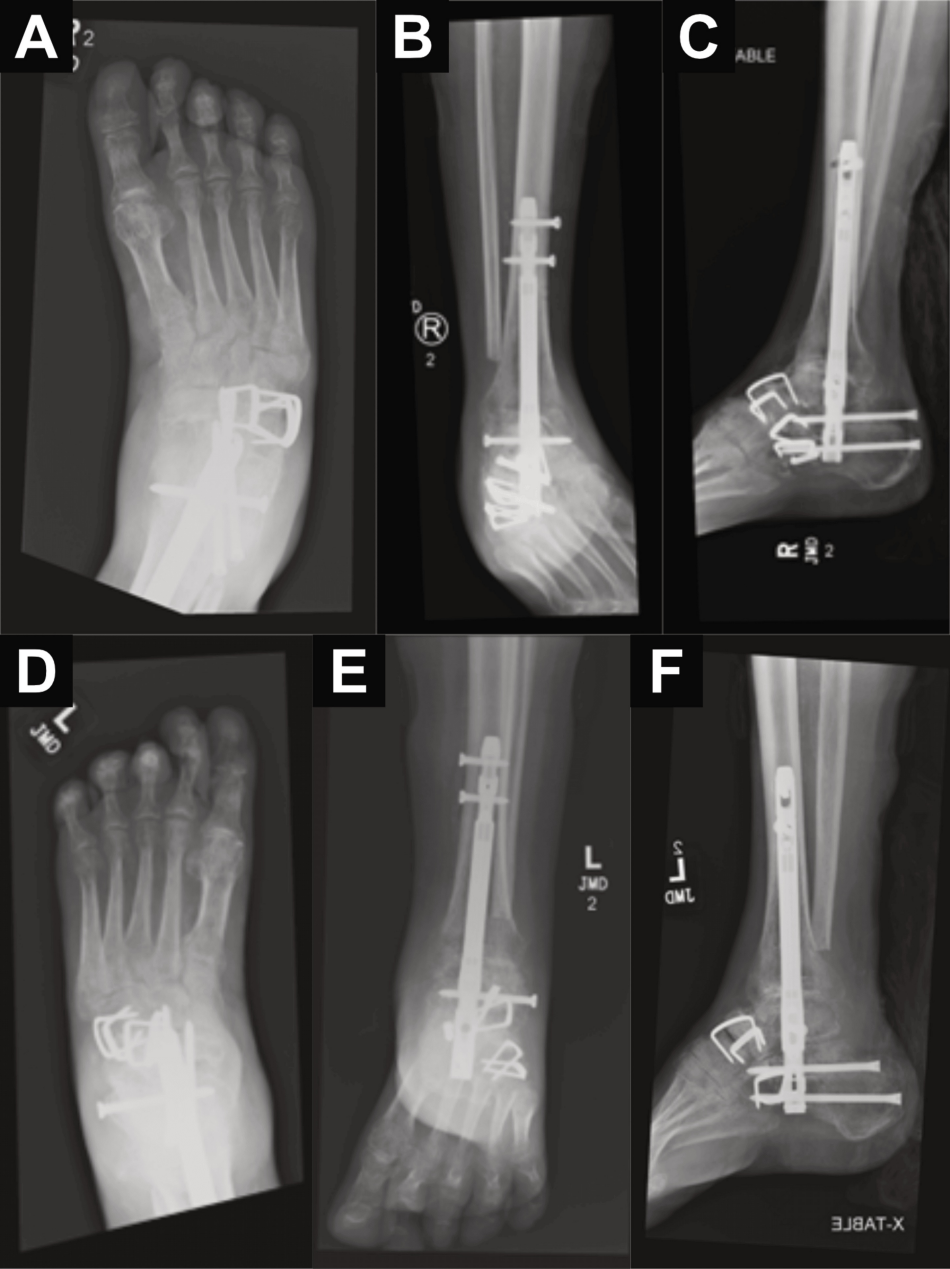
Nine-month postoperative radiographic views following bilateral pantalar arthrodesis A: Right foot anteroposterior view, B: right ankle anteroposterior view, C: right ankle lateral view, D: left foot anteroposterior view, E: left ankle anteroposterior view, F: left ankle lateral view
